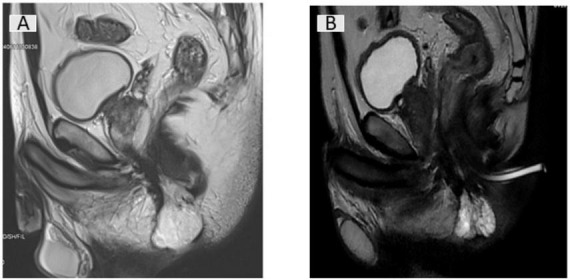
Sagittal T2-weighted pelvic MRI before (A) and after (B) chemoradiotherapy.
